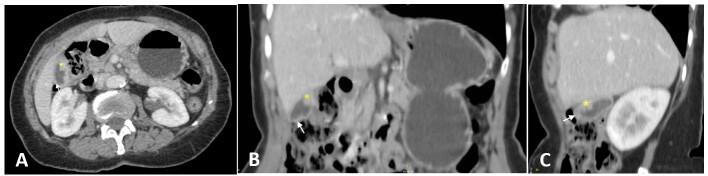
Abdominal Computed Tomography scan shows generalized thickening of the gallbladder wall (white arrows) and the polypoid and solid components (yellow marks) in axial (A), coronal (B), and sagittal (C) planes.
